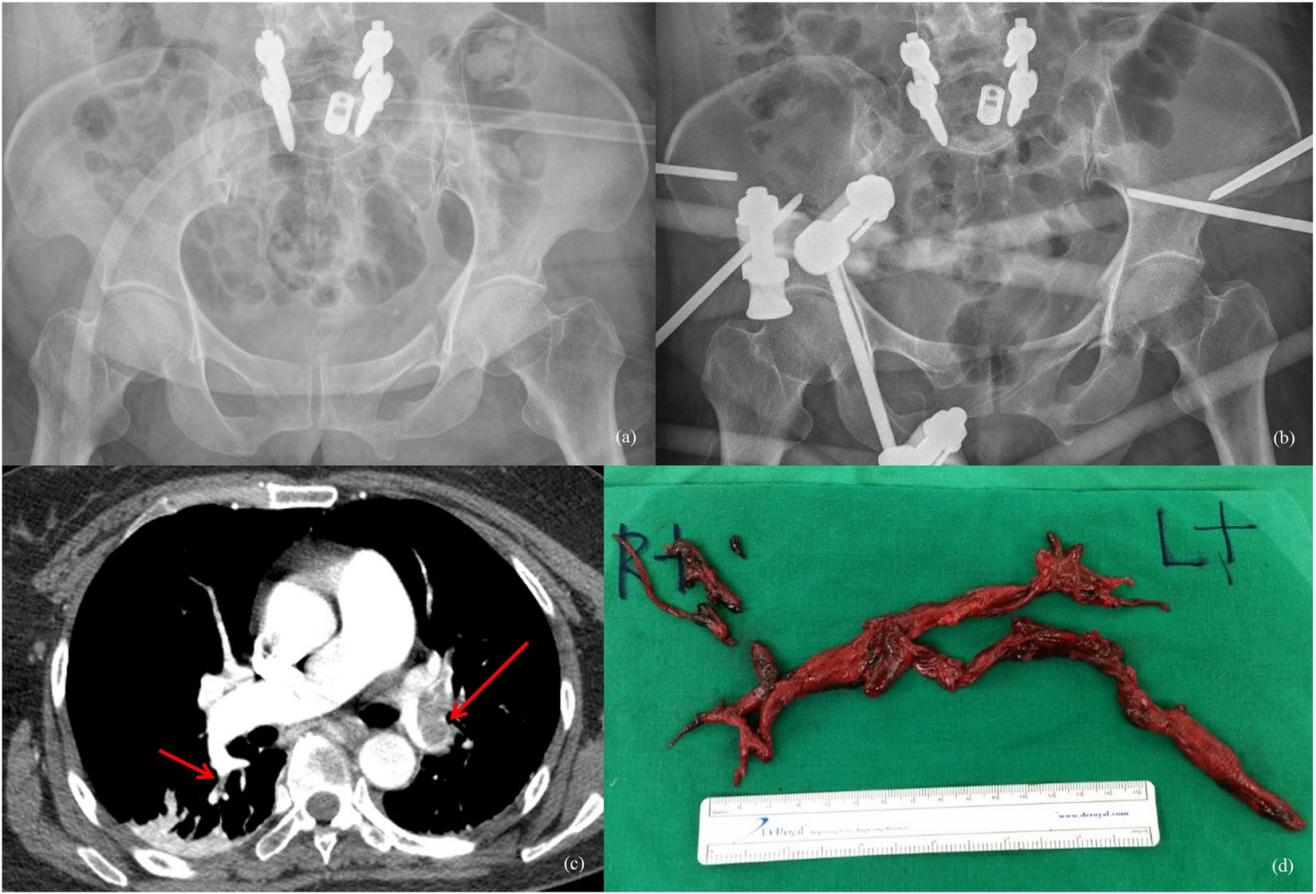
A 52-year-old female patient with a left rami fracture and diastasis of the left SI joint after a traffic accident underwent general anaesthesia for pelvic ring stabilization (a). After general anaesthesia, the patient rapidly developed hypoxia and tachycardia and the operation was not performed. Only external fixation was performed (b). CT angiography was performed to confirm total occlusion of the left pulmonary artery and right segmental thrombus (red arrow) (c). Thrombectomy was immediately performed (d).
